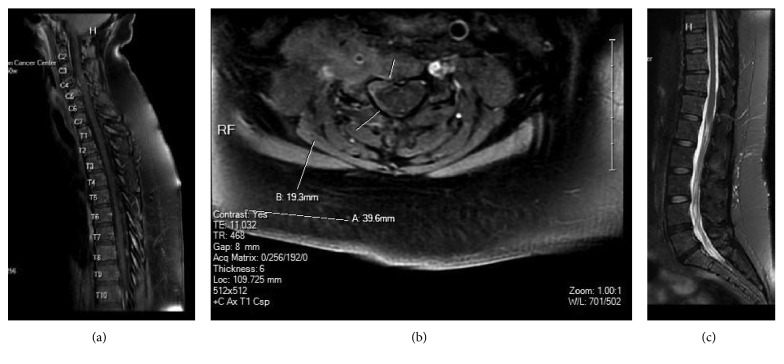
(A and B) MRI cervical and thoracic showed C5 metastasis that extended into the right C4-C5 and C5-C6 foramina (C) with leptomeningeal metastasis.
